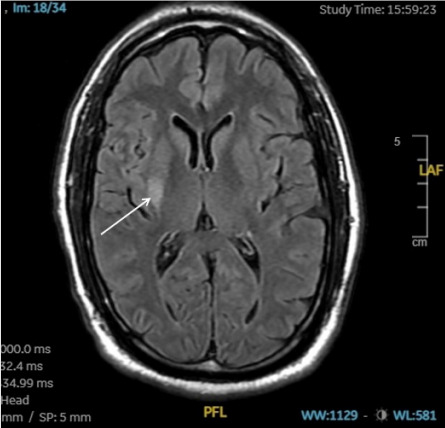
T2 FLAIR of magnetic resonance imaging brain revealing an acute ischemic infarct in the right basal ganglia and insular region (white arrow). *FLAIR*, fluid-attenuated inversion recovery.
